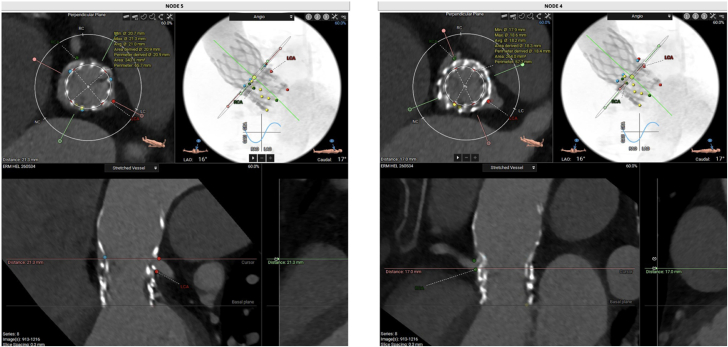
Computed Tomography Angiography-Based Measurements Including Estimation of Potential New Skirt Planes at Nodes 4 and 5 and Area Measurement The new skirt plane at node 4 was 21.3 mm and was chosen for safe deployment below the coronary risk plane. The area at node 4 was 264 mm^2^. CTA = computed tomography angiography.
